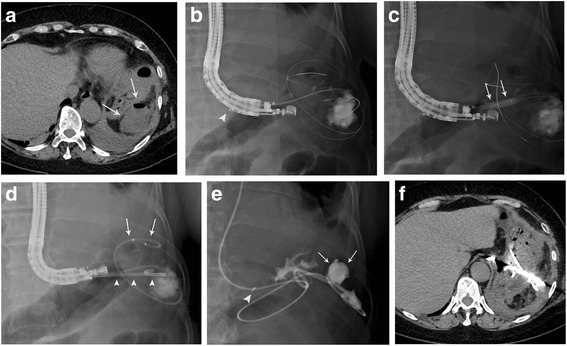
a CT shows accumulated fluid containing gas (arrows) in the left subphrenic area adjacent to the fornix of the stomach. b Fluoroscopy image shows the guidewires coiling in the abscess cavity. The hemoclip (arrowhead) is placed at the esophageal junction to avoid transesophageal puncture. c Fluoroscopic image shows an 8-mm-diameter balloon catheter (arrows) dilating the ostomy between the stomach and the abscess cavity. d A 5-cm-long 7F double pigtail stent (arrowheads) and a 6F naso-abscess catheter (arrows) are placed into the cavity. e Fluoroscopic image with contrast medium enhancement via the naso-abscess external catheter shows shrinkage of the abscess cavity (arrows). The hemoclip (arrowhead) is placed at the esophageal junction. f CT demonstrates the drainage catheter and stent, and reduction of the abscess cavity
